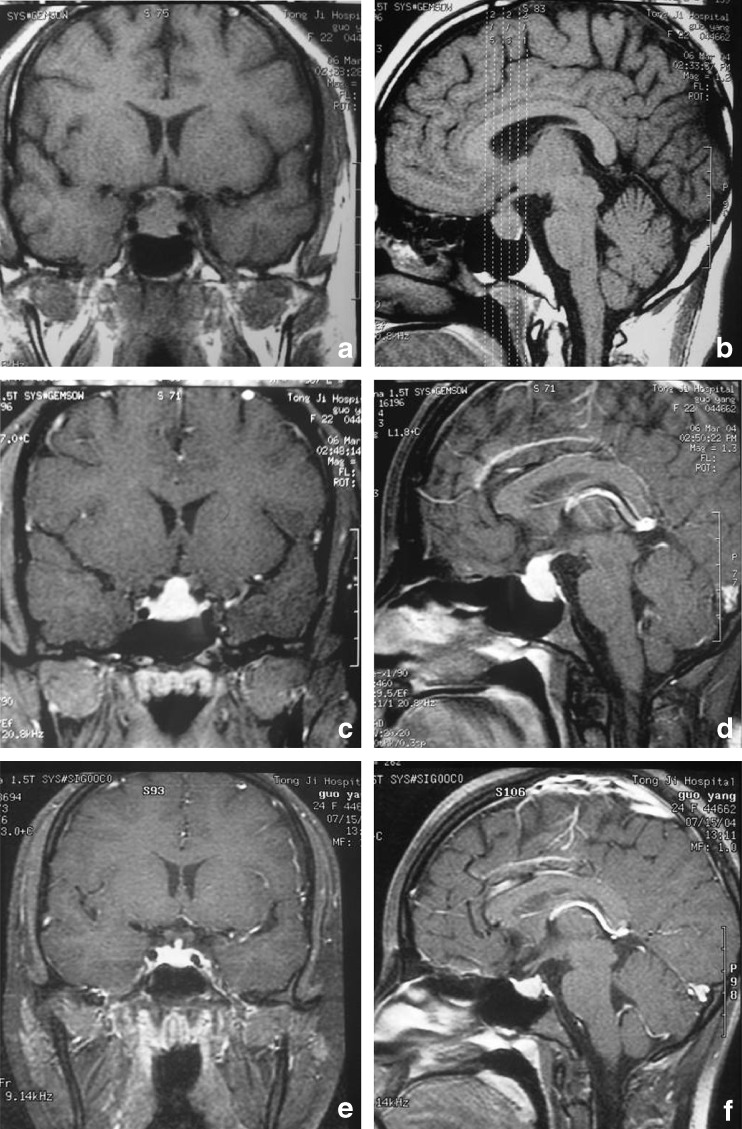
a, b MRI coronal scan showing pituitary enlargement with equal T1 signal, close to the optic chiasm. c, d MRI scan showing a pituitary mass obviously and uniformly enhanced by Gd-EDTA injection. e and f MRI coronal, sagittal scan showing pituitary size return to normal size after taking thyroxine tablets
